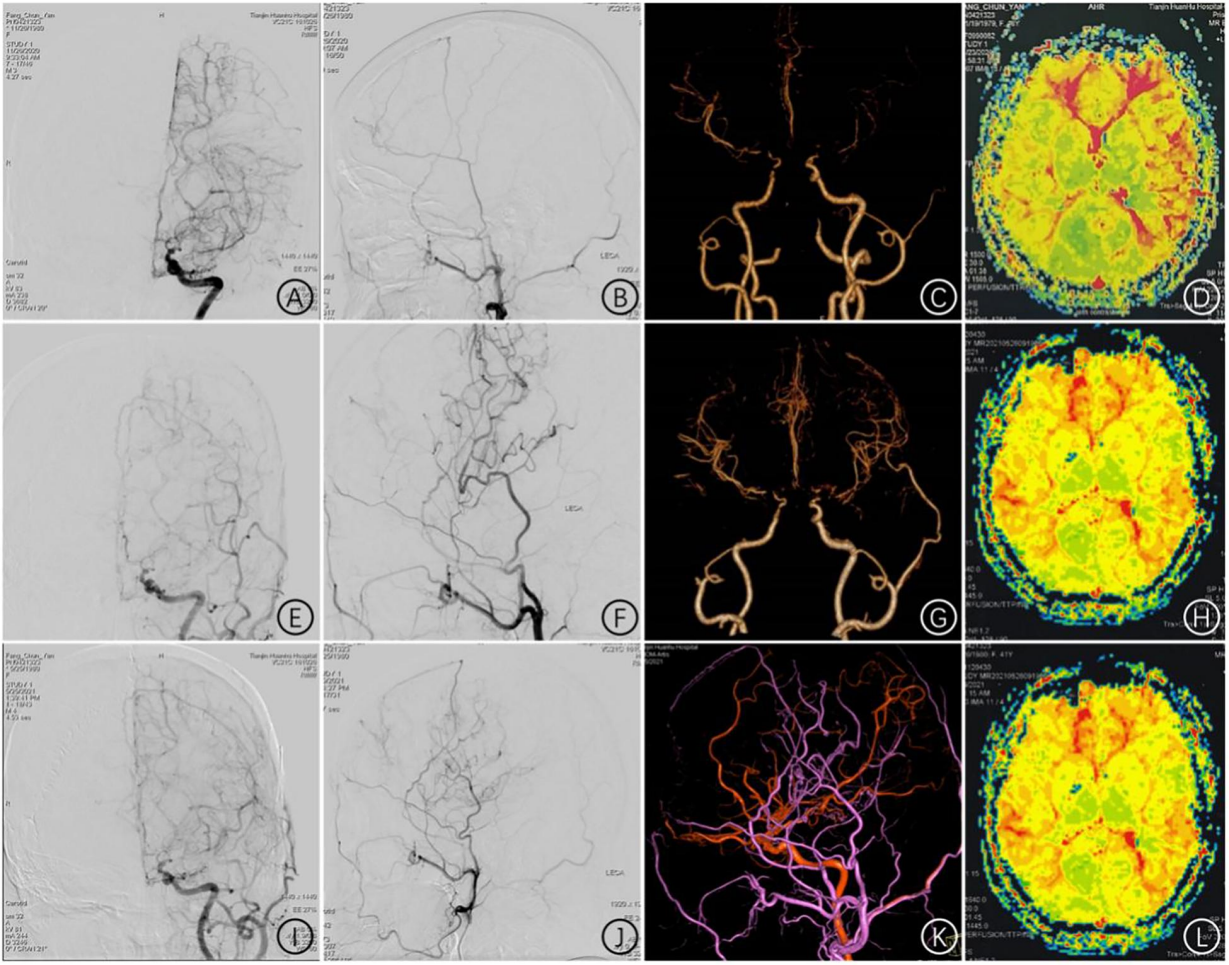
Imaging follow-up of cases of STA-MCA + pedicled temporoparietal fascia application combined with blood flow reconstruction surgery (A) preoperative DSAT indicated stenosis at the end of the left internal carotid artery and smoke vessel formation; (B) preoperative DSA lateral angiography of the left external carotid artery; (C) preoperative CTA showed stenosis at the ends of bilateral internal carotid arteries; (D) preoperative PWI indicated hypoperfusion in the left cerebral hemisphere; (E,F) angiography of the left external carotid artery 5 days after the operation indicated unobstructed STA-MCA; (G) CTA 10 days after the operation indicated that the STA-MCA was unobstructed; (H) postoperative PWI indicated an improvement in hypoperfusion of the left cerebral hemisphere; (I,J,K) angiography reexamination 6 months after the operation indicated that the left STA-MCA remained unobstructed; (L) six months after the operation, PWI indicated improved perfusion in the left cerebral hemisphere.
